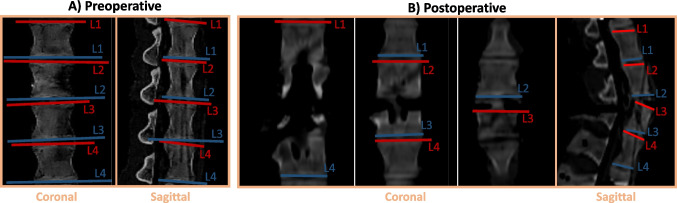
Manual spinal alignment assessment on preoperative (A) and postoperative (B) CT scans. Superior endplates are shown in red color, while inferior endplates are shown in blue. Vertebra identification allows to make the correspondence between the endplate and the vertebra it belongs to
